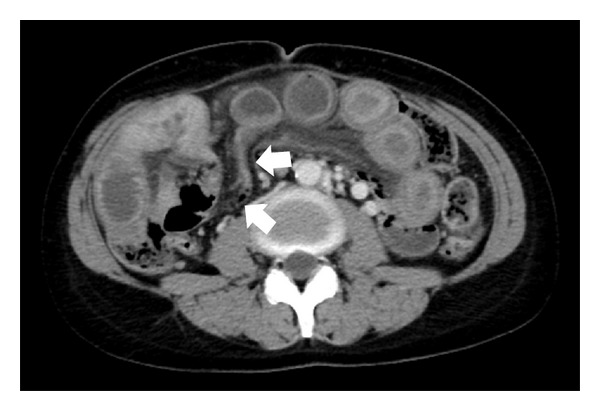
Abdominal computed tomography before surgery. Computed tomography demonstrated internal hernia of the small intestine (arrow: small intestine through the hernia orifice).
